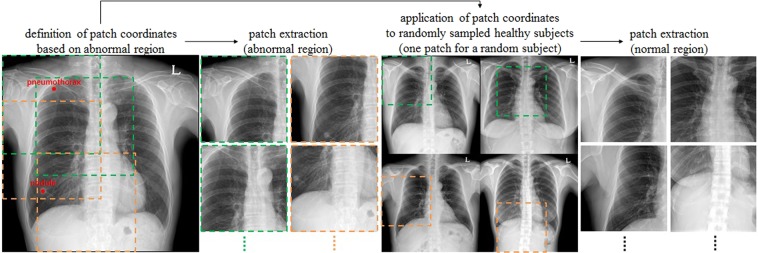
Lesion-based patch extraction for the curriculum learning in chest X-rays (CXRs).
